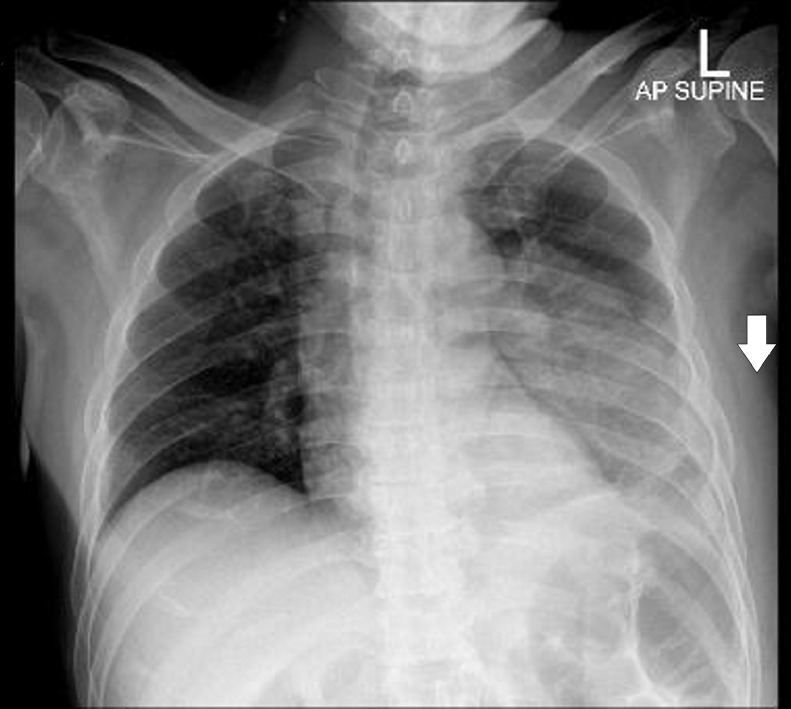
Chest radiograph of the initial presentation, showing consolidation occupying the middle and the lower zone of the left lung with left pleural effusion; thickening of the left thoracic wall's soft tissue (white arrow).
